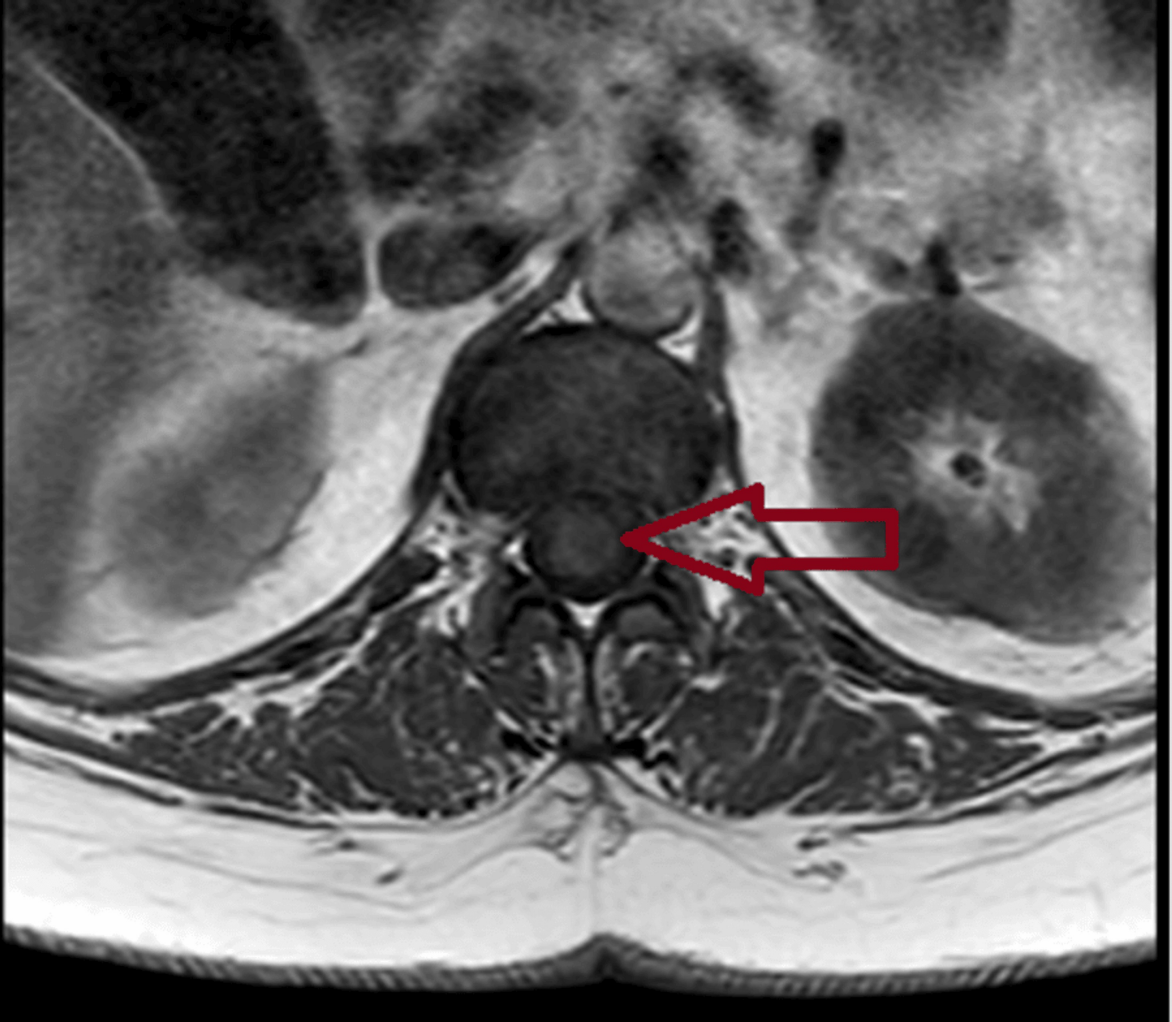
Axial T1‑weighted MRI at the conus level demonstrating intramedullary signal abnormality consistent with acute spinal cord infarction
